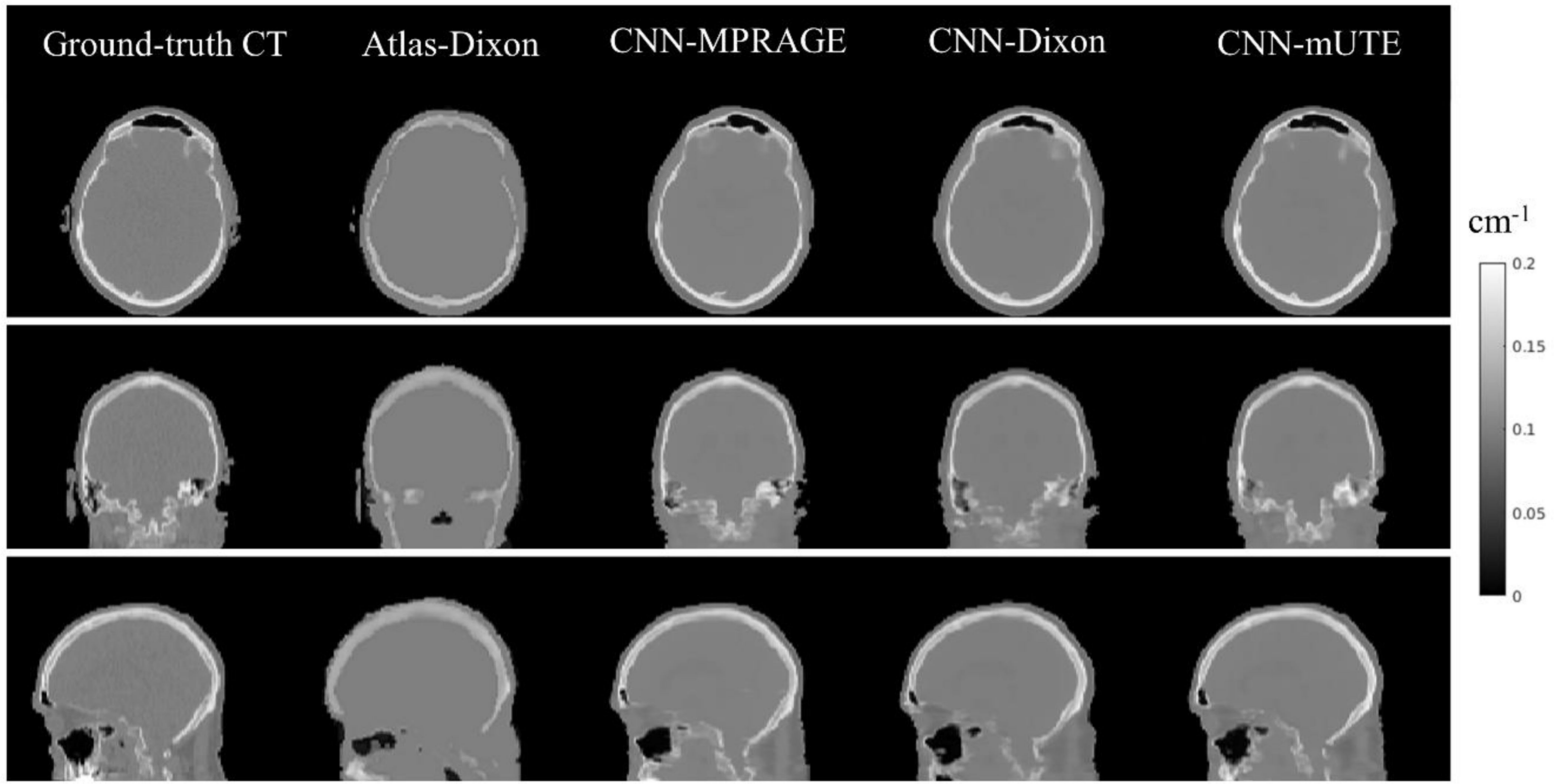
Three views of the ground-truth CT and the generated pseudo-CT images of one subject using different methods: Ground-truth CT (first column), Atlas-Dixon (second column), CNN-MPRAGE (third column), CNN-Dixon (fourth column) and CNN-mUTE (fifth column).
